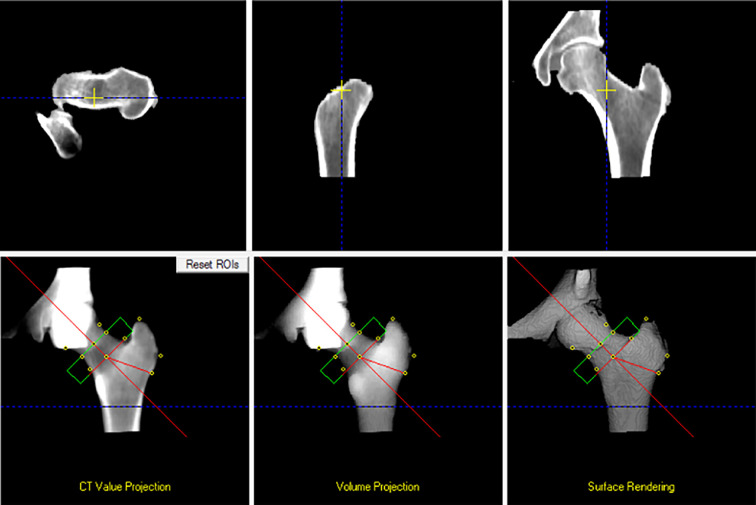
3D reconstruction and ROI placement for bone mineral density measurement. Multiplanar views (CT value projection, volume projection, and 3D surface rendering) showing the automatic placement of standard ROIs (femoral neck, intertrochanteric, and total hip) used for calculating *T*-score and %BMD according to ISCD guidelines.
